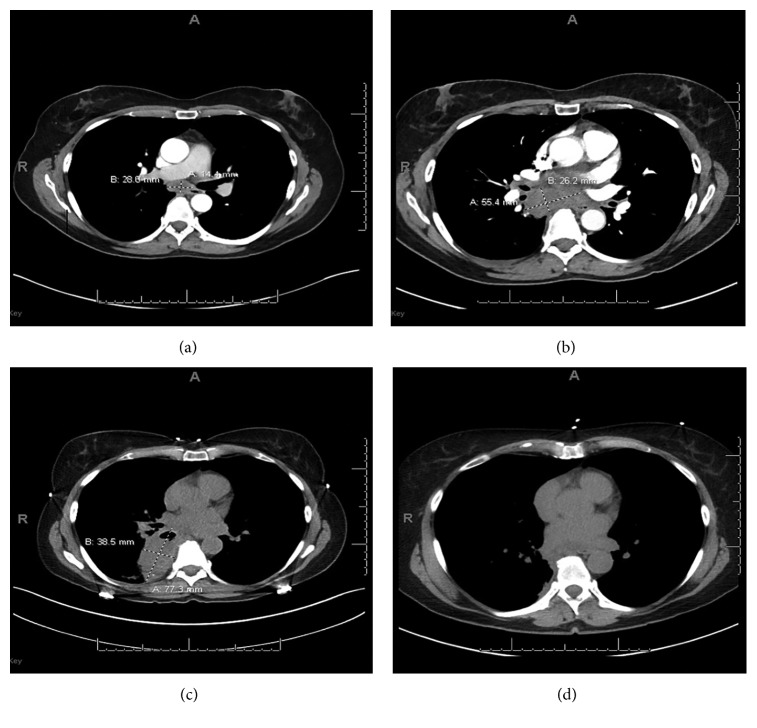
(a) Initial chest CT with newly identified lymphadenopathy. (b) Chest CT 10 days after EBUS with new subcarinal mass. (c) Chest CT 26 days after EBUS with right lower lobe pulmonary abscess. (d) Chest CT following a 6-week course of IV and oral antibiotic therapy (mediastinal and lung windows).
